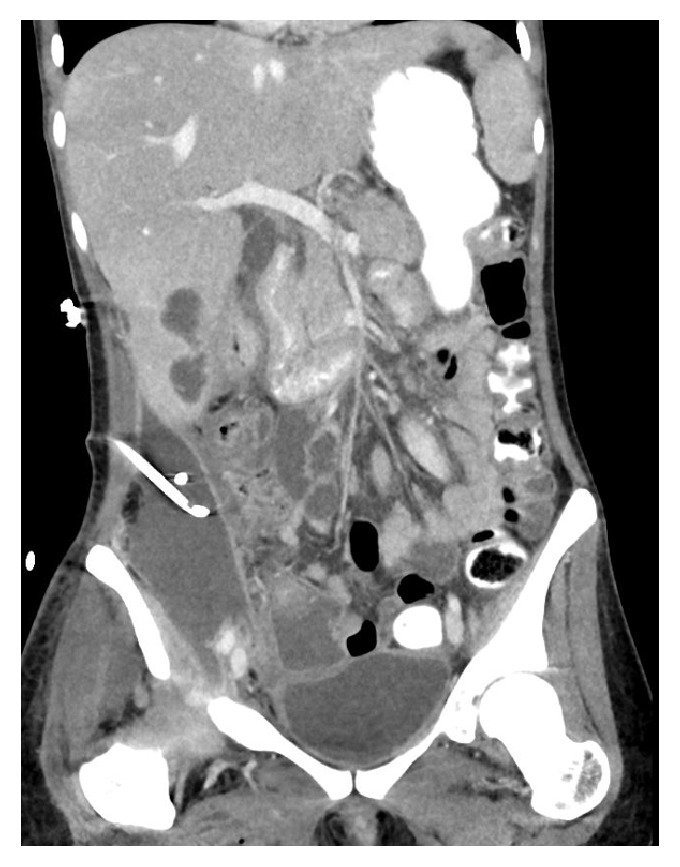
Computed tomography shows the retroperitoneal abscess after the insertion of the transcutaneous drainage.
